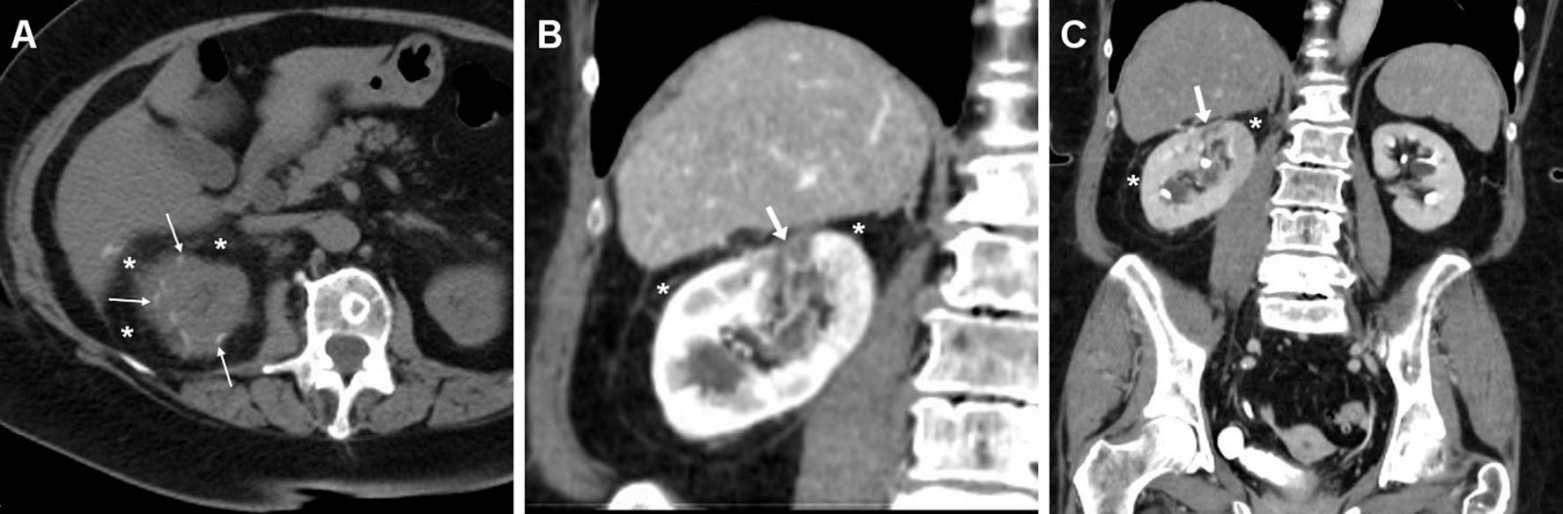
Normal early postoperative imaging appearance in a 77-year-old woman investigated with multidetector CT 4 days after laparoscopic enucleation of a 2-cm RCC of the right kidney. Unenhanced axial image (a) showed hyperattenuating linear structures (*thin arrows*) corresponding to surgical sutures at the upper renal pole, and normal peri-renal fat (*). Axial nephrographic (b) and coronal excretory-phase (c) images showed a focal, wedge-shaped, non-enhancing portion of the renal cortex (*arrows*) with continuous renal contour corresponding to the resection site, and confirmed normal peri-renal fat planes (*) without extravasated blood and urine. Despite postoperative pain and blood loss, the patient had an uneventful postoperative course and was discharged without further treatment
